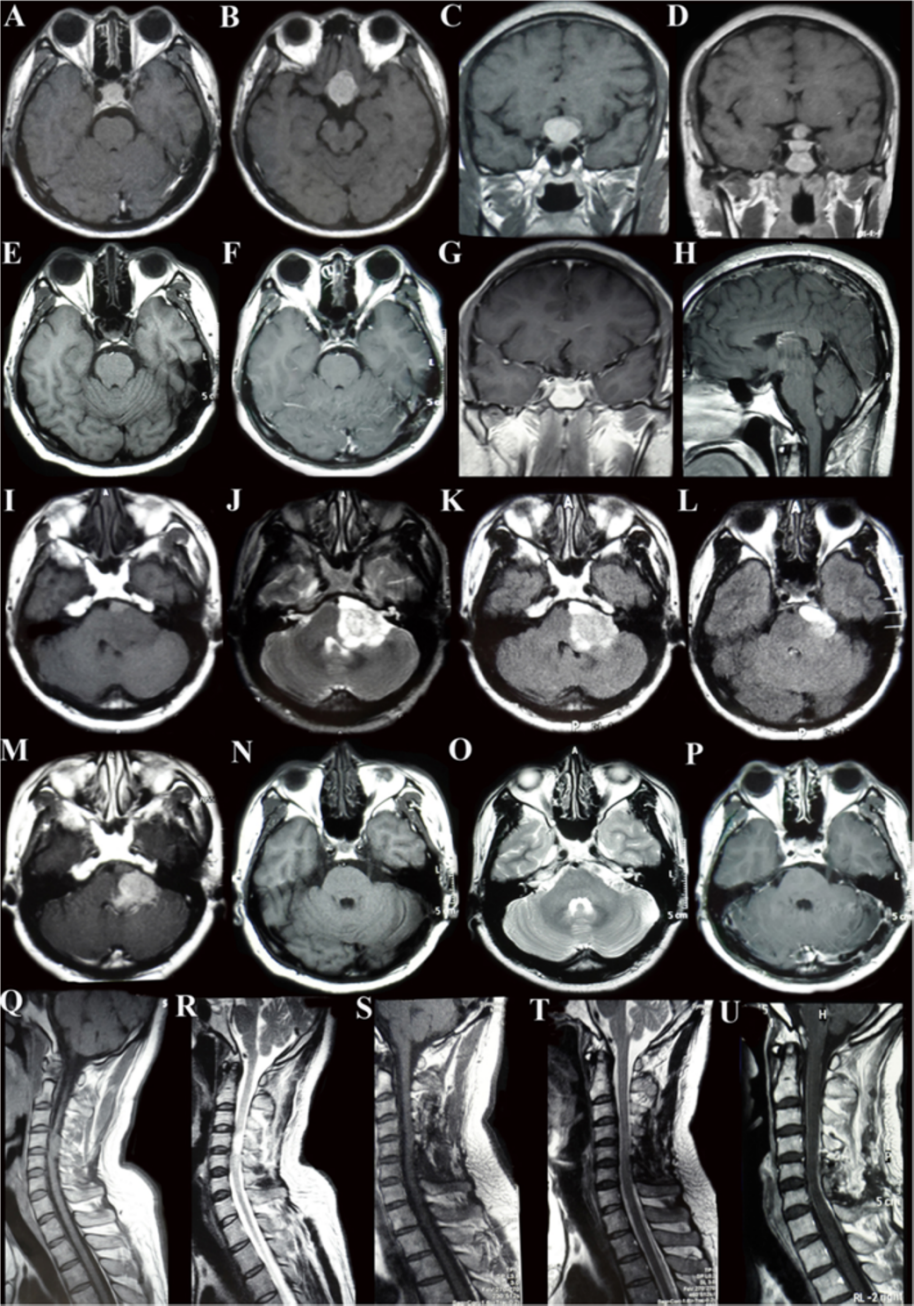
Pre- and postoperative magnetic resonance imaging scans from the first three surgeries.
